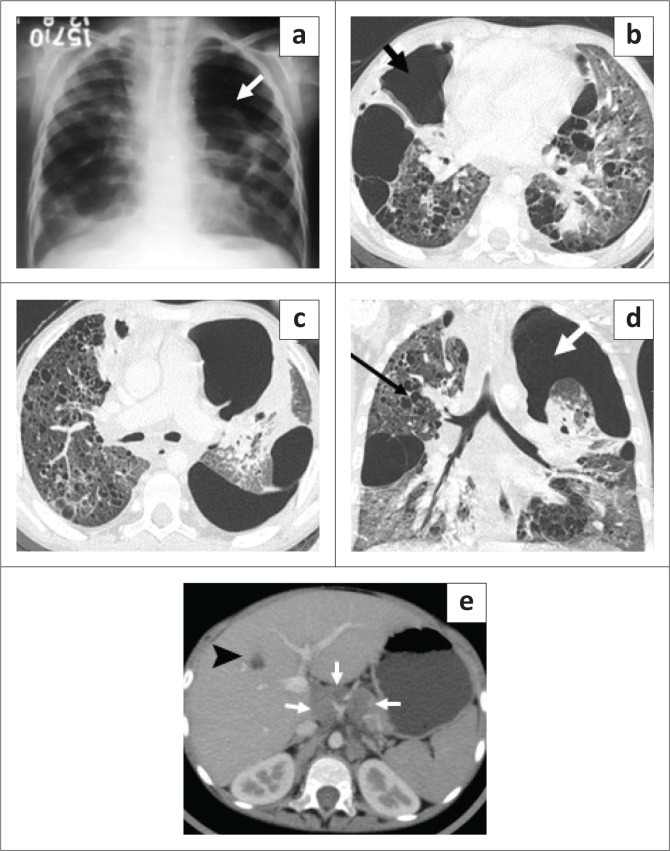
A 7-year-old boy with disseminated TB on antitubercular therapy. Chest radiography (CXR) (a) shows a loculated left pneumothorax (arrow) and cystic lucencies in both lungs. Contrast-enhanced CT chest axial (b, c) and coronal (d) images show a left pneumothorax (white arrow), cavitation (short black arrow) and innumerable variable-sized cysts on both lungs (long arrow) with a few ground glass opacities. Axial section of the abdomen (e) revealed multiple enlarged periportal, paraaortic and coeliac lymph nodes (arrows) and a hepatic granuloma (arrowhead).
